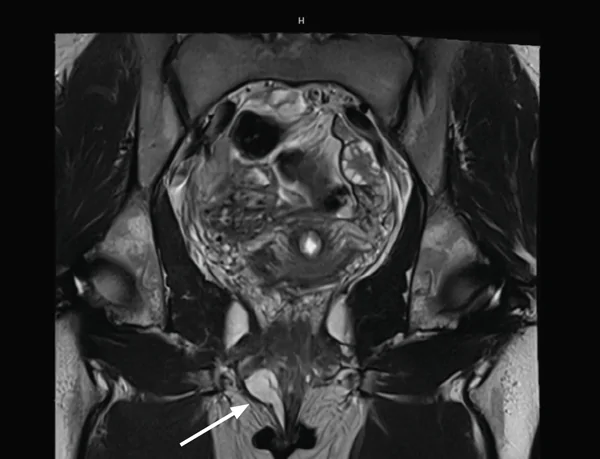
Canal of Nuck cyst as seen on MRI, incorrectly characterized as Bartholin′s cyst abscess.
